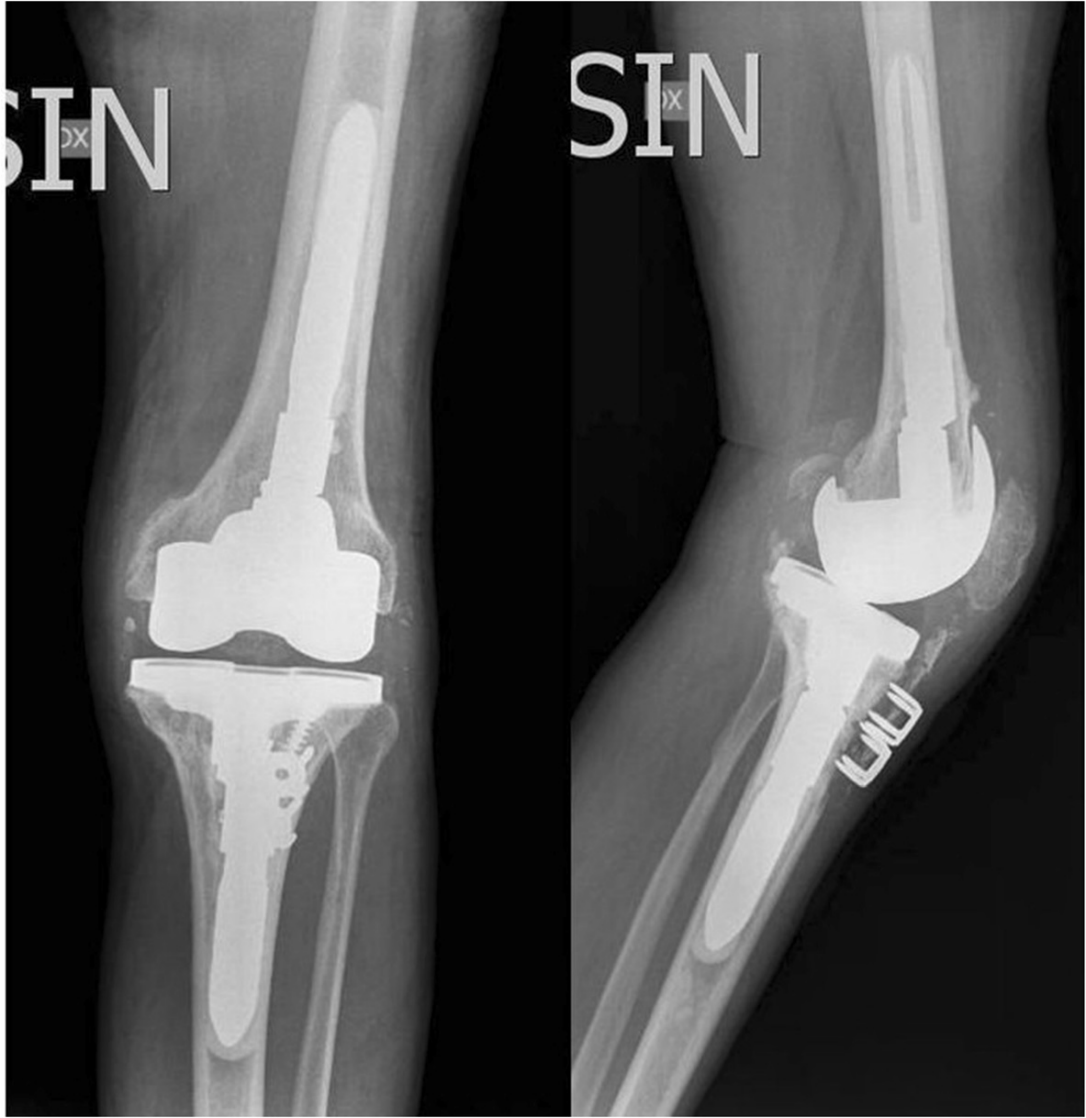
Post-operative radiographs following the TKA revision without resurfacing the patella and 2 staples used to fix the medially detached tibial tubercle.
